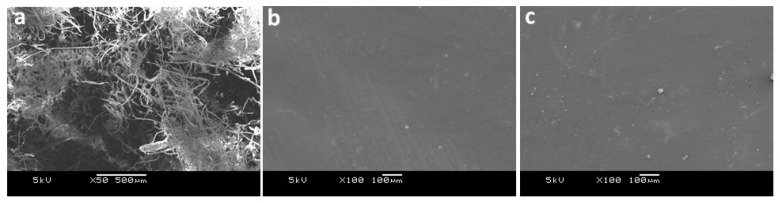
SEM images of (a) cellulose, (b) CTA-1 and (c) CTA-2.
